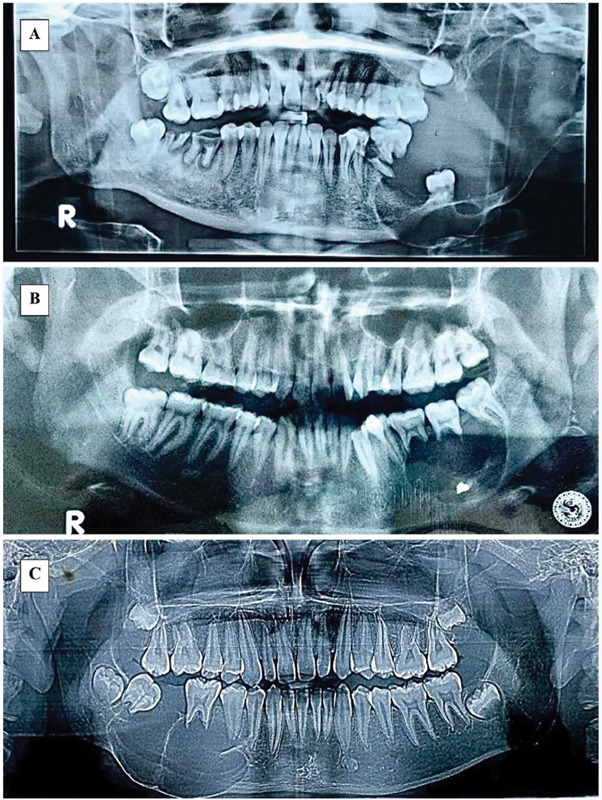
Radiograph examination of ameloblastoma. (
